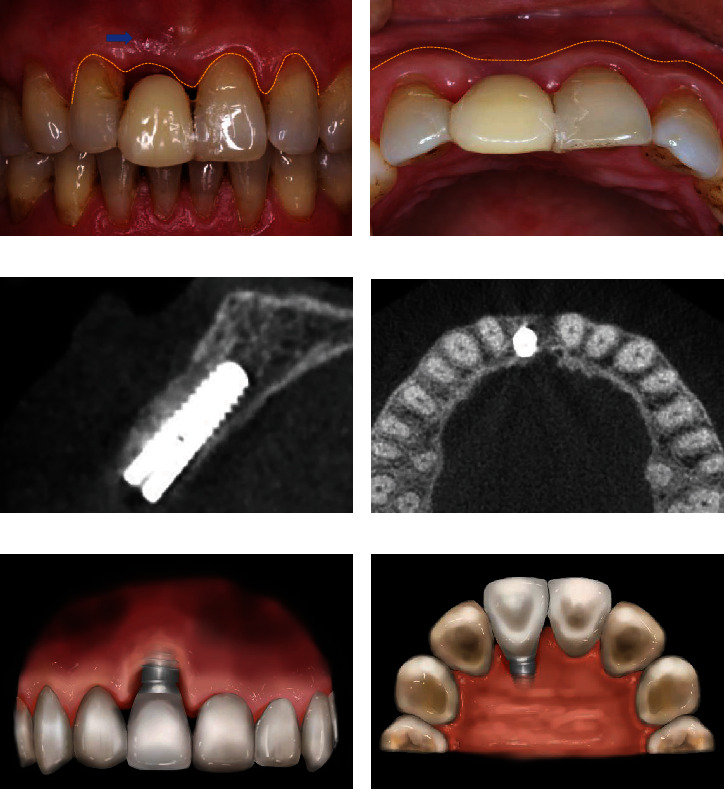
Clinical and radiographic views 3 months postoperative of immediate implant placement. (a) The labial view showing a fistula and papillary recession mesial and distal to the 11. (b) The occlusal view displaying labial peri-implant soft tissue contour collapse. (c, d) The radiographic view 3 months postoperative of implant placement exhibiting no radiolucent lesion around the implant and no resorption of the peri-implant bone. (e) The labial view showed soft tissue recession of TTSD. (f) The lingual view showed soft tissue recession of TTSD.
